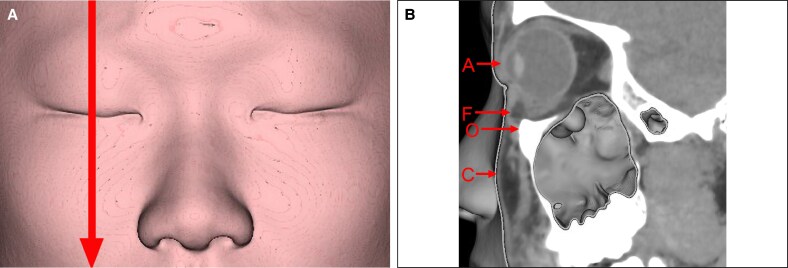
Parameter measurements from computed tomography scans. (A) For each patient, a 3-dimensional reconstruction of the soft tissue was generated, and the mid-pupillary plane on the left side (vertical line) was identified. (B) The orbital view was then aligned with the mid-pupillary plane and visualized from a standard left lateral perspective. The soft tissue reconstruction provided clear visualization of the anterior surface of the globe (Point A), the anterior margin of the inferior orbital fat pad (Point F), the infraorbital rim (Point O), and the most anterior projection of the cheek (Point C).
